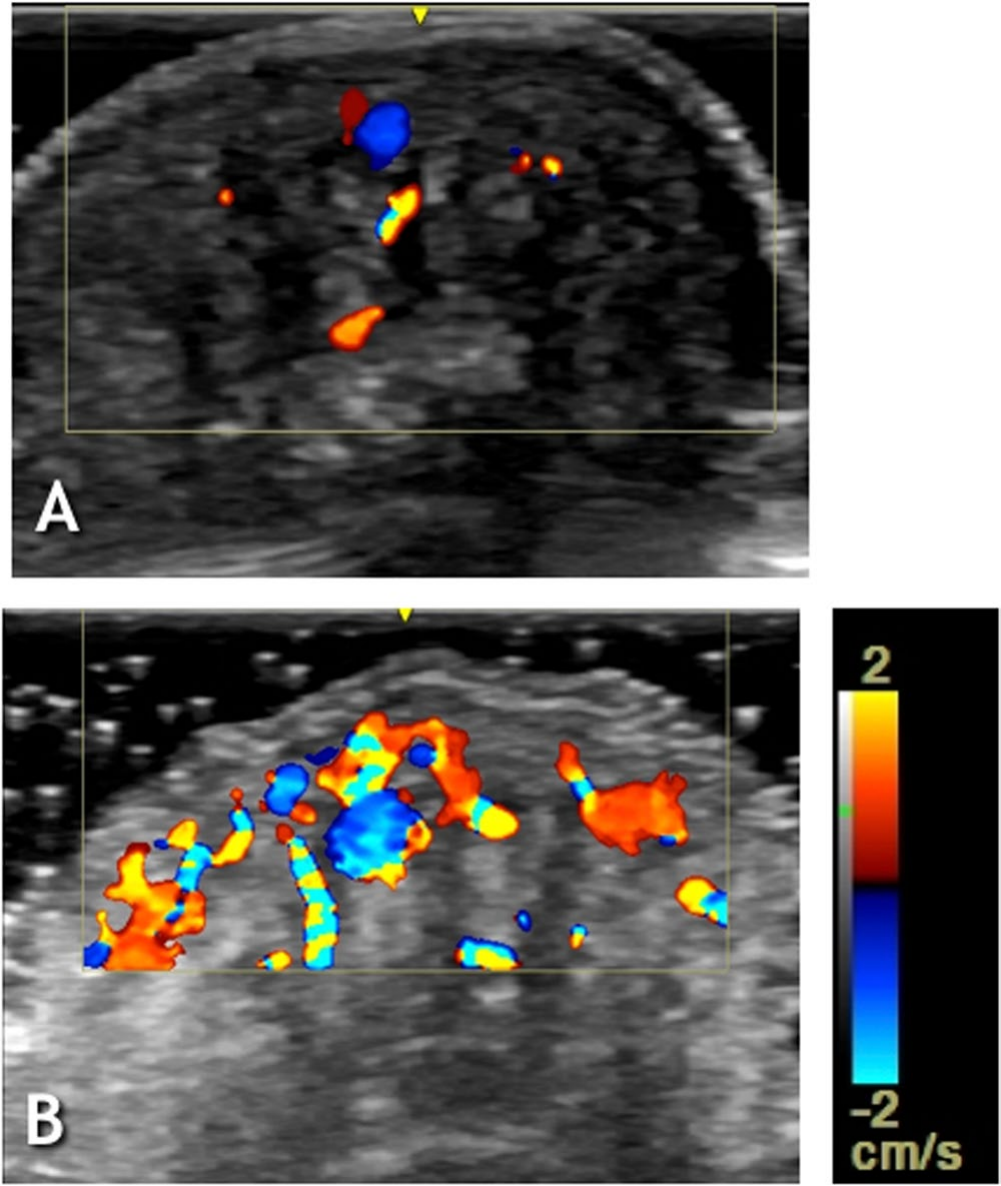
Index finger – transverse view. (A) US and Power Doppler Imaging Before WISW. (B) US and Power Doppler Imaging After WISW. Please note increased color flow signal after WISW in the dermis and hyper-echogenic areas.
